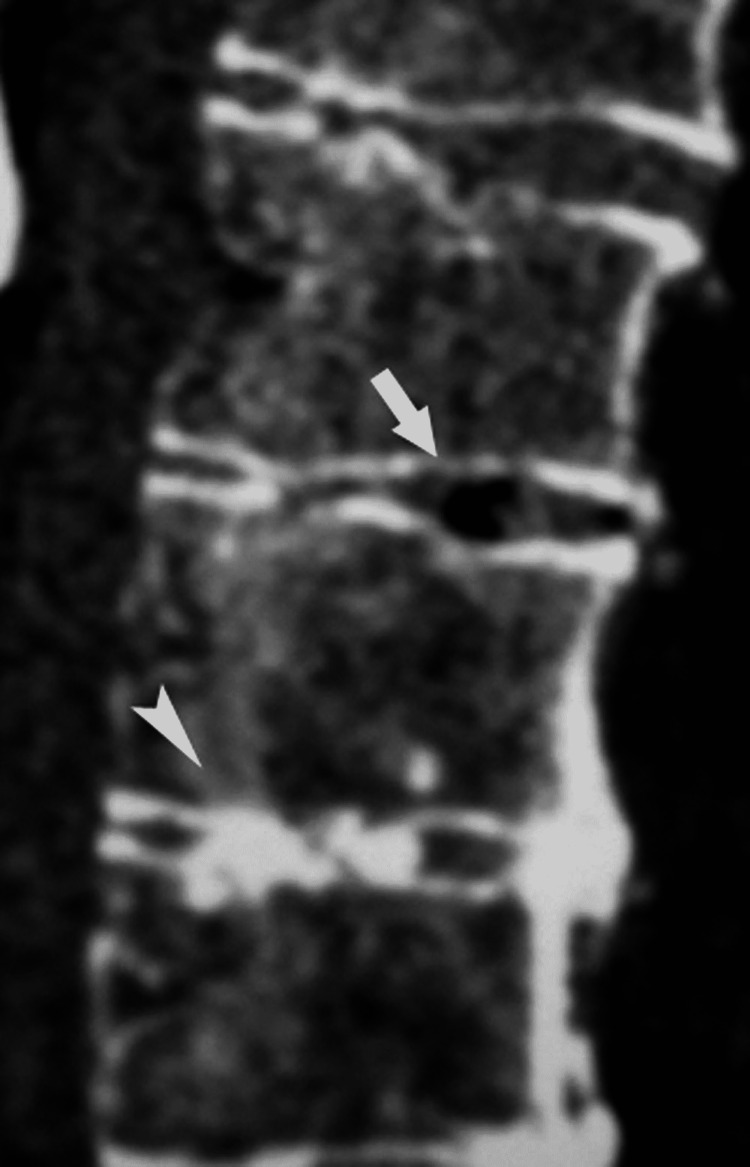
Vacuum disc. A reformatted sagittal CT image reveals a radiolucent area consistent with a vacuum phenomenon in the disc (arrow). Note the calcification of the upper disc (arrowhead).
